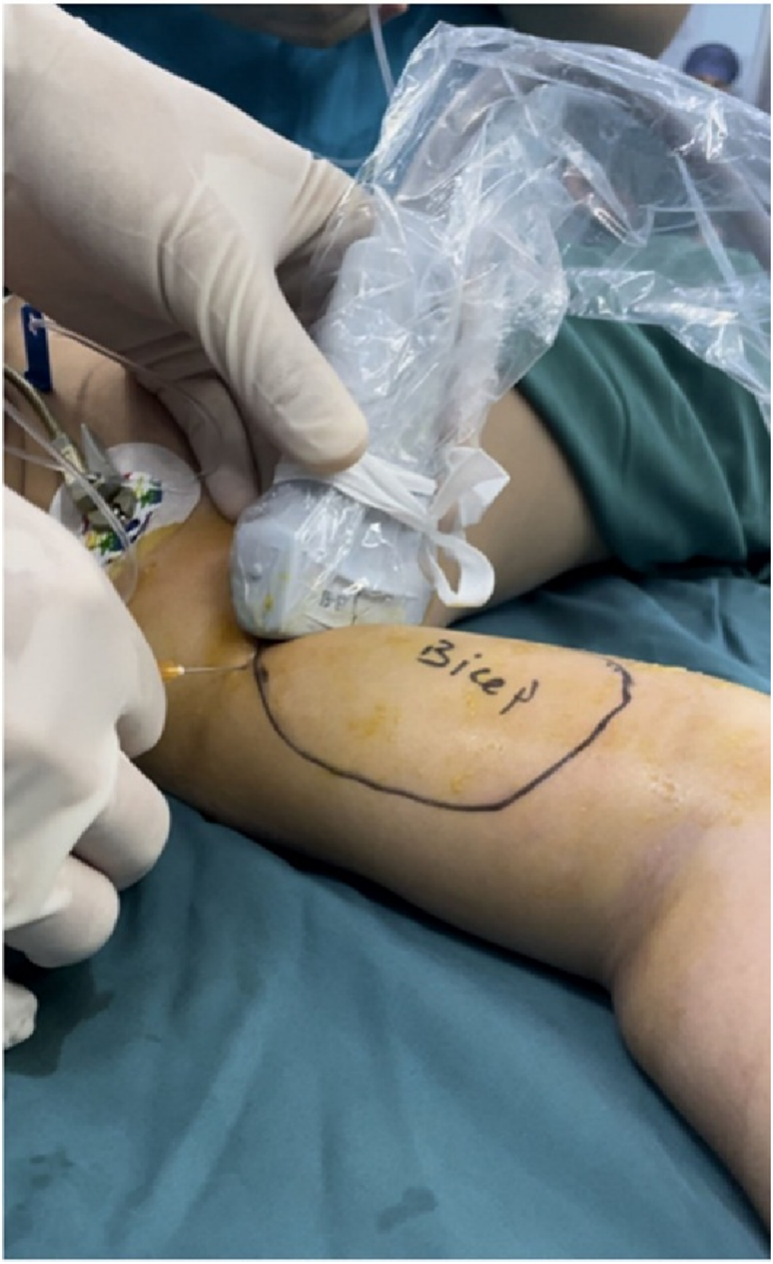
General image of ultrasound-guided coracobrachialis plane musculocutaneous nerve block.
